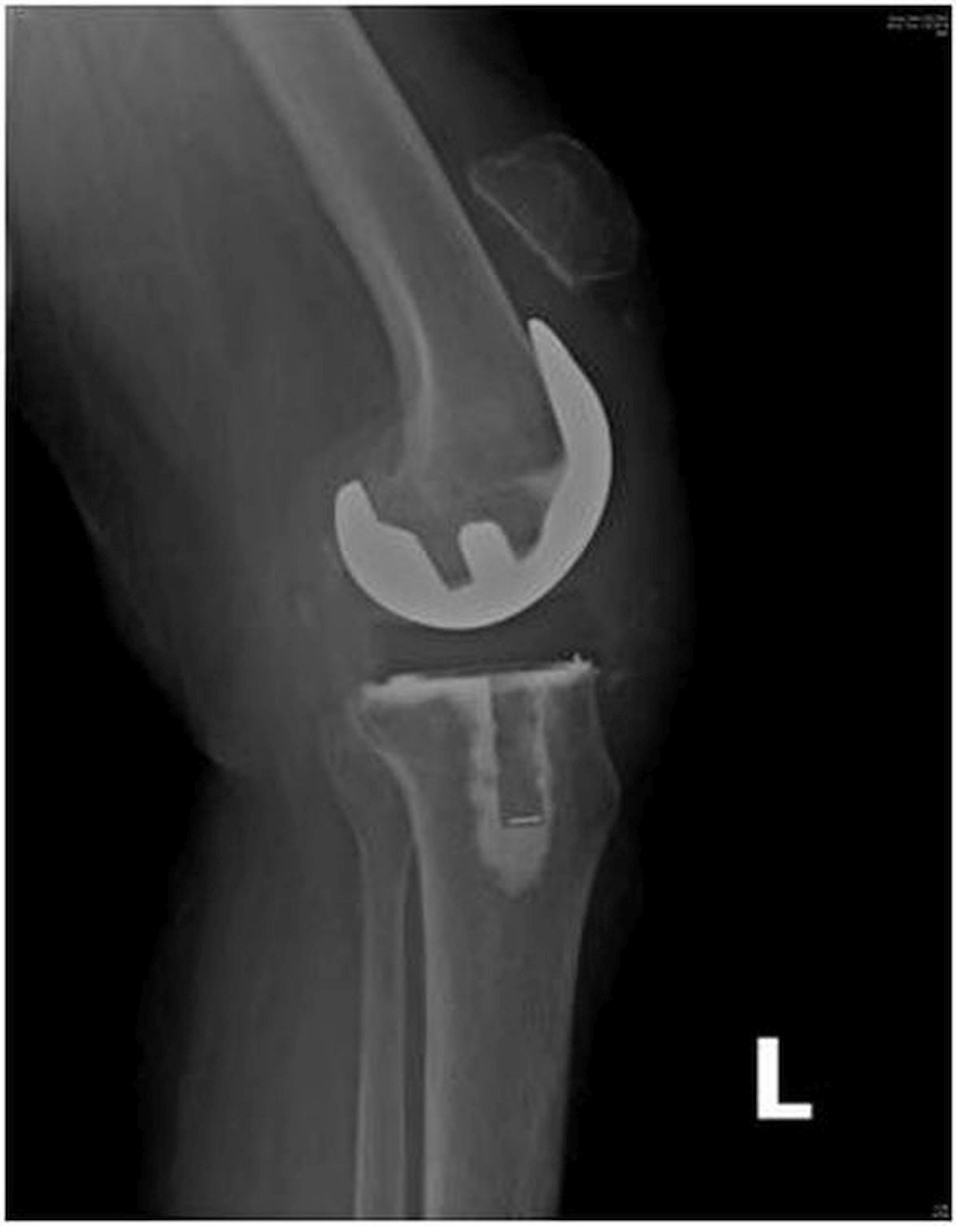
Lateral radiograph of a patient showing an infrapatellar tendon rupture and proximal migration of the left patella.
